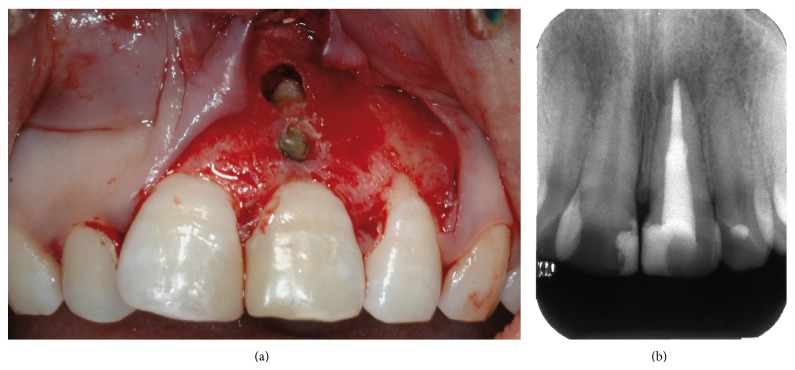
(a) Curettage of the lesions after raising the split thickness flat. (b) Radiograph taken during the surgery.
